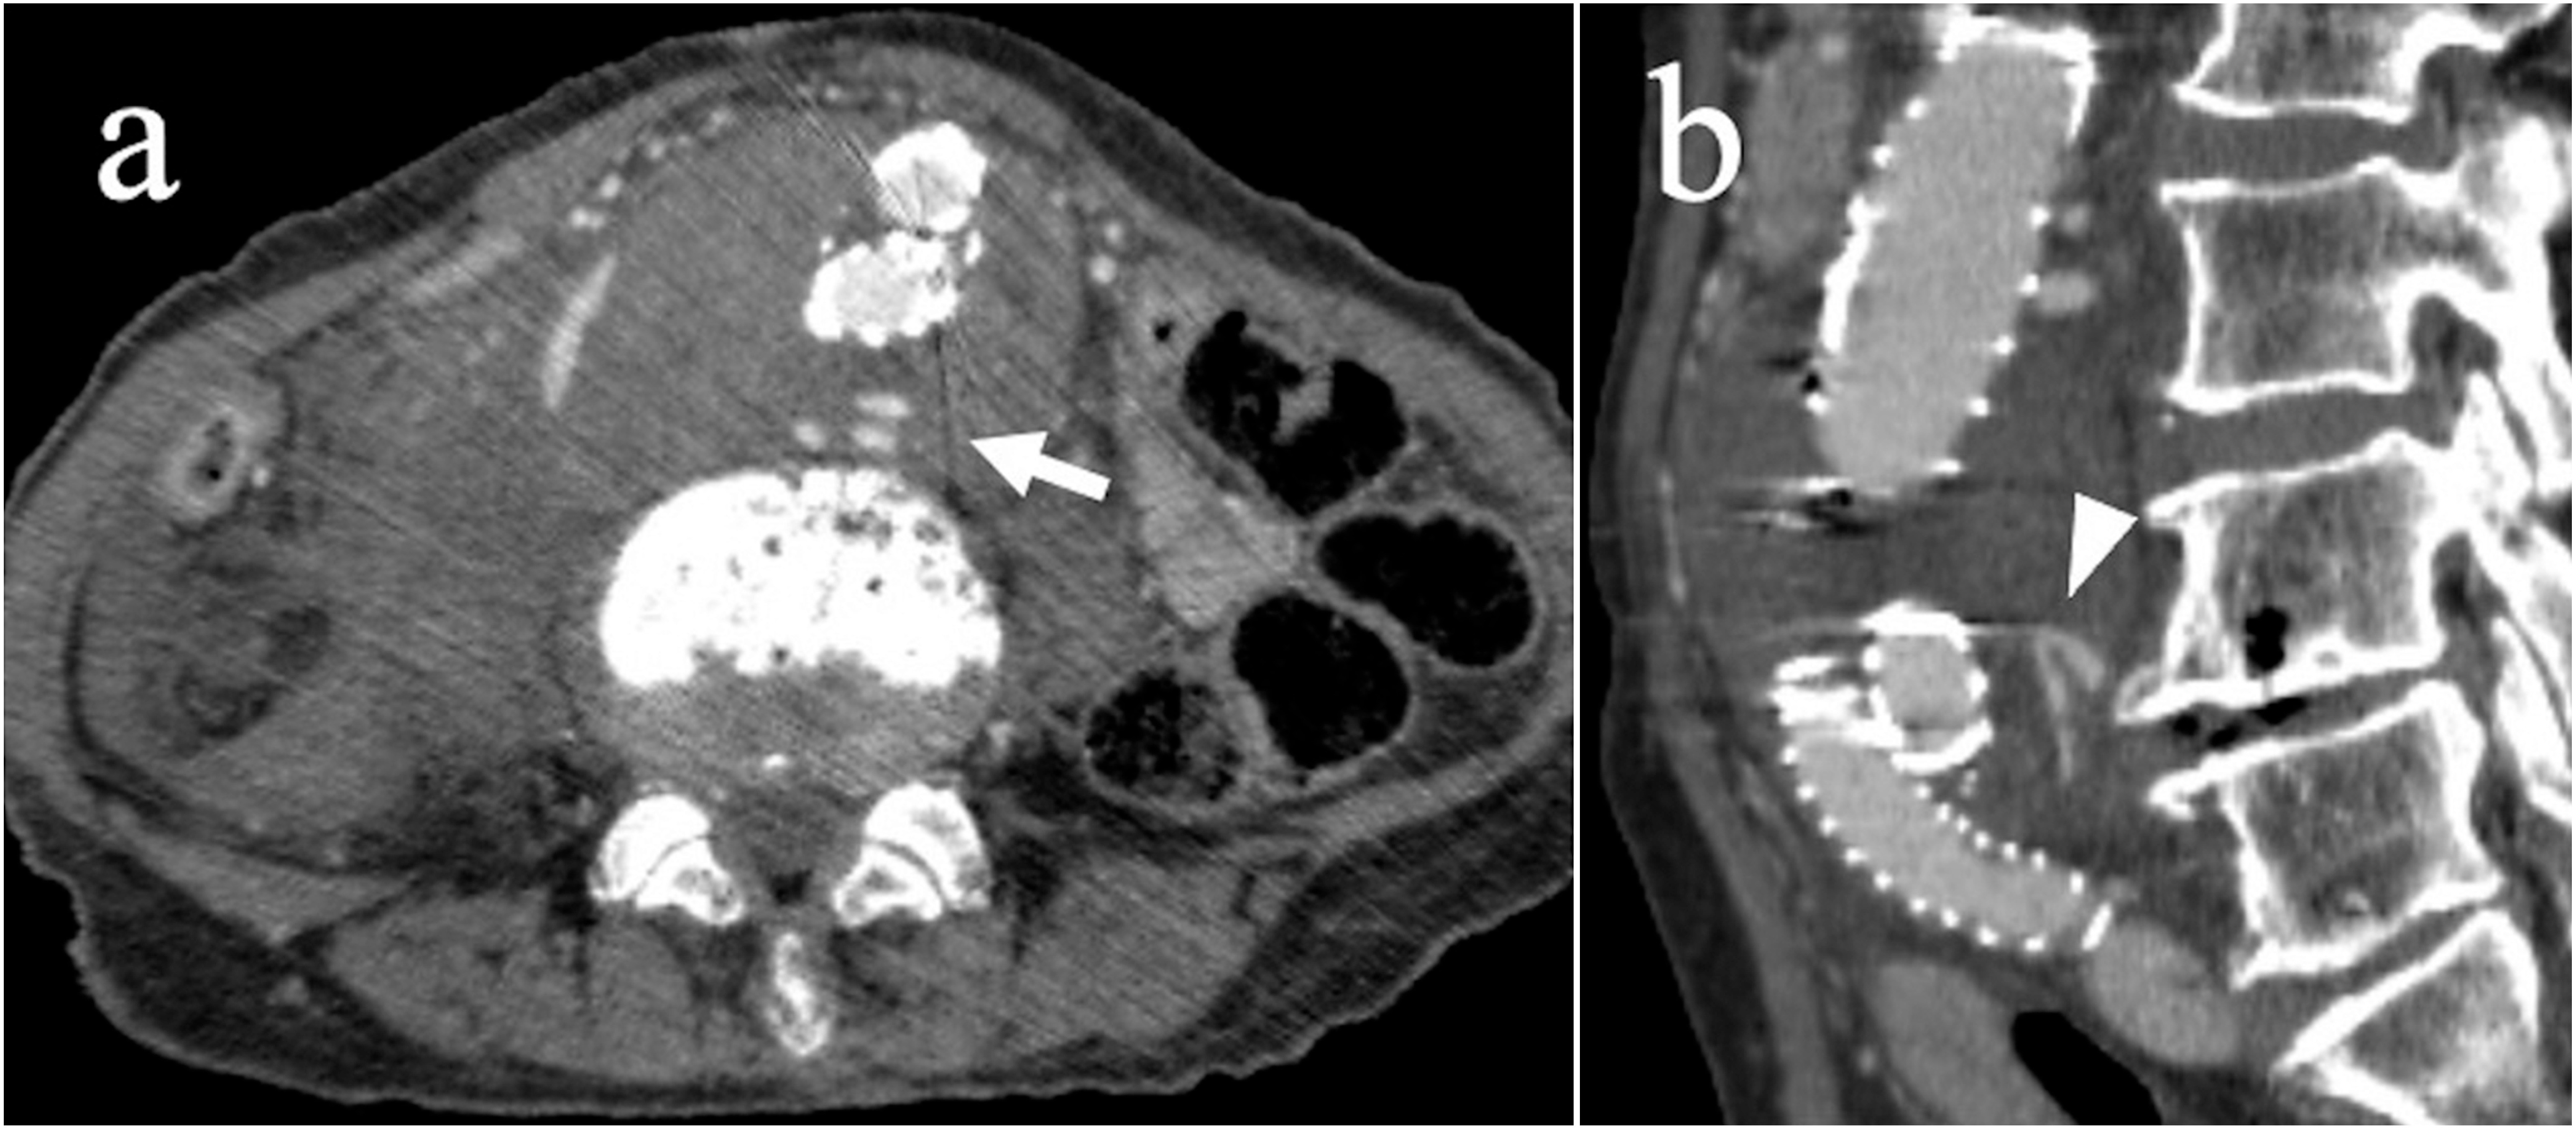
Fig. 1 (a) Axial view of CT imaging shows retroperitoneal hematoma around the sac of abdominal aortic aneurysm and extravasation of contrast media (white arrow). (b) Sagittal view of CT imaging shows a sharp spike formation on the lumbar vertebra adjacent to the area of extravasation (white arrowhead).
